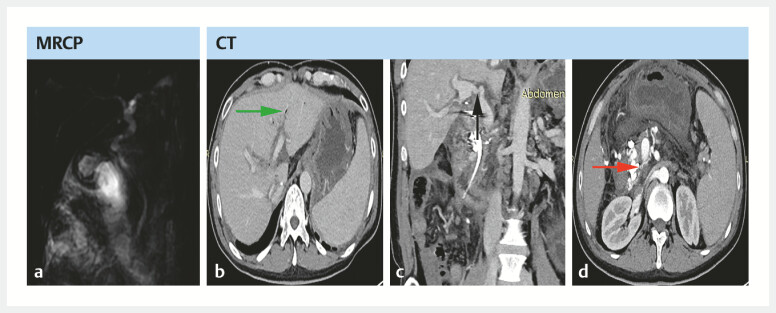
CT and MRCP before the third ERC.
